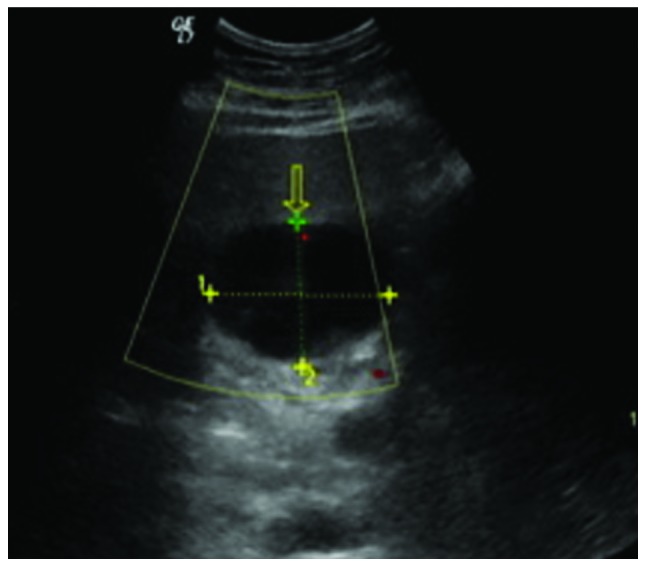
Abdomen Doppler ultrasound. A well-defined hypoechoic area (~53×39 mm) at the quadrate lobe of the liver, showing that the internal echo of the mass was heterogeneous.
